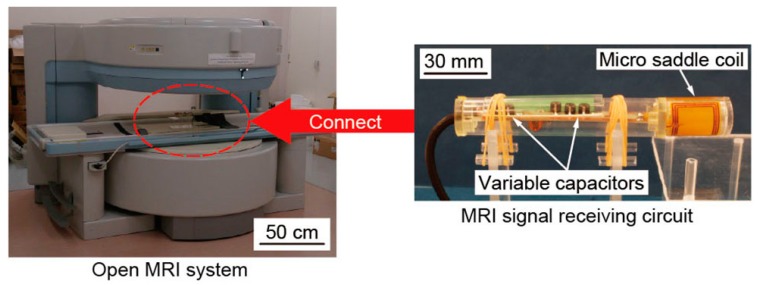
Experimental setup for measuring the MRI images. The micro saddle coil is attached to the receiving circuit, which is connected to the MRI system.
